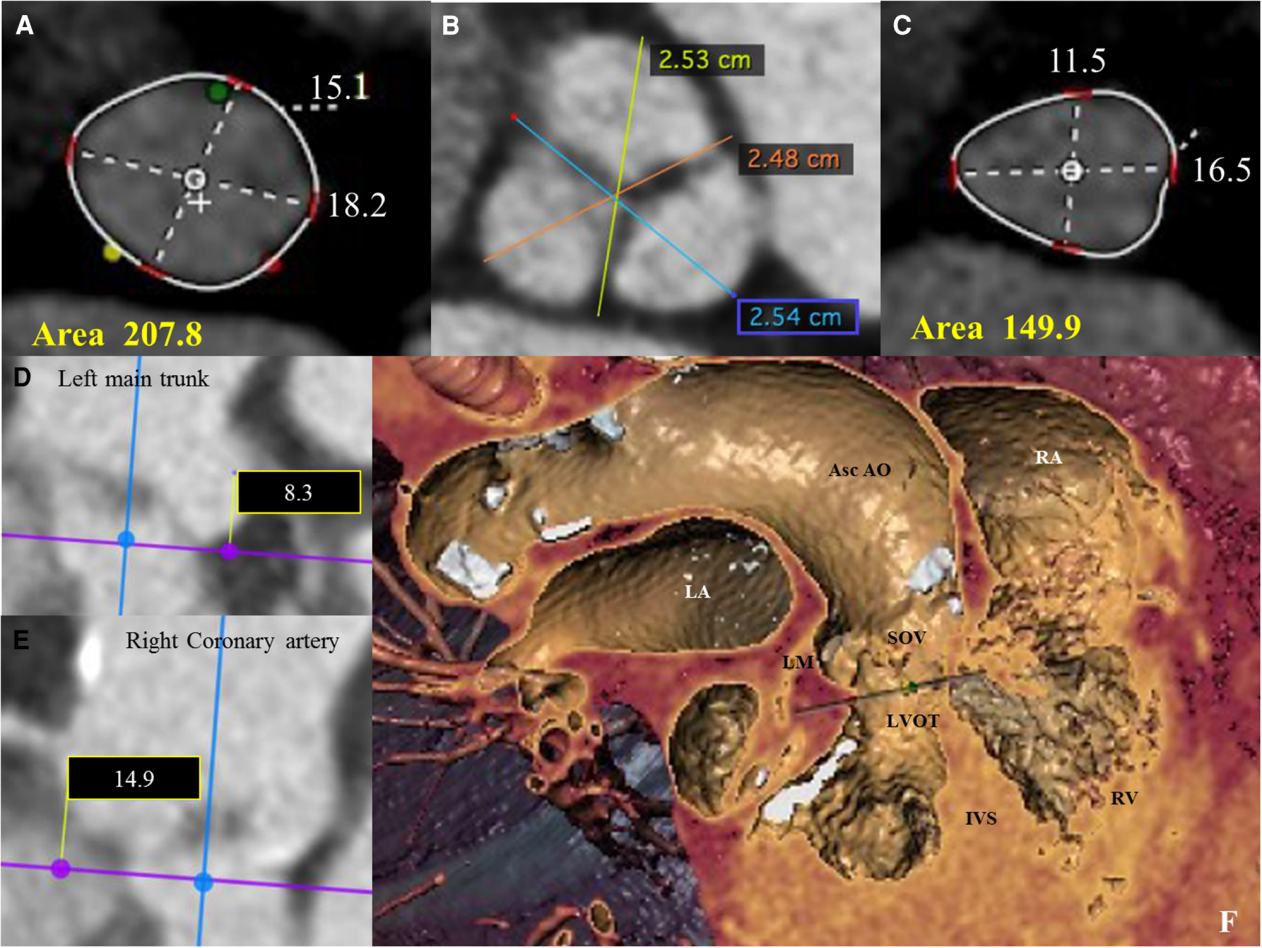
(A) Pre-operative thoraco-abdominal computed tomography (CT) images were analyzed using 3mensio Structural Heart software (Pie Medical Imaging, Netherlands): the aortic annulus perimeter was 51.7 mm (minor annulus diameter 15.1 mm, major annulus diameter 18.2 mm) and the area was 207,8 mm^2^. (B) Pre-operative thoraco-abdominal CT images showed a tricuspid aortic valve without significant valvular calcifications (images reconstructed with OsiriX DICOM Viewer Pixmeo SARL, Switzerland). (C) Pre-operative thoraco-abdominal CT images showed a LVOT perimeter of 45.3 mm with elliptical shape (minor annulus diameter 11.5 mm, major annulus diameter 16.5 mm) and an area of 149,9 mm^2^ (images reconstructed with 3mensio Structural Heart software). (D and E) CT images showing the distance from the virtual basal ring (VBR) to left main trunk of 8.3 mm (see D) and to the right coronary artery of 14.9 mm (see E). (F) Pre-operative thoraco-abdominal CT images showing the heart chambers and the ascending aorta according to the ‘double oblique virtual reality’ reconstruction with 3mensio Structural Heart software. Asc Ao, Ascending Aorta; IVS, interventricular septum; LM, left main coronary trunk; LA, left atrium; LVOT, left ventricular outflow tract; RA, right atrium; RV, right ventricle.
